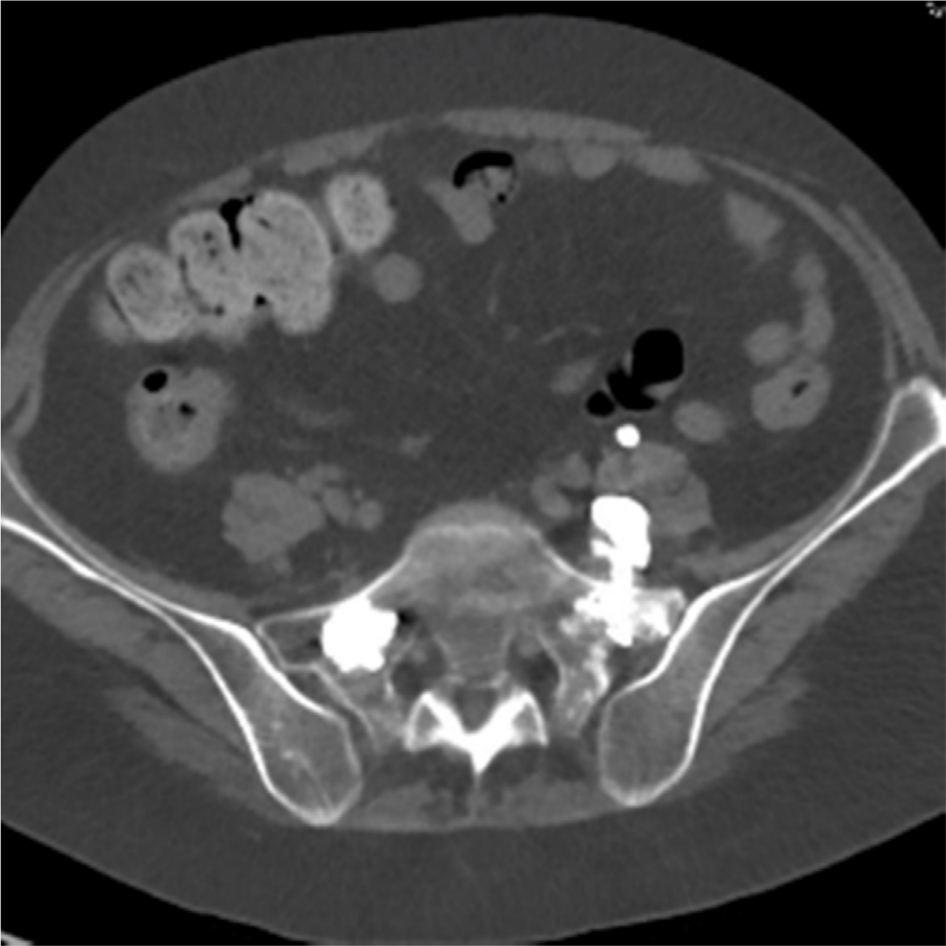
Axial view CT without contrast of lumbosacral spine demonstrating cement extravasation anterior to left sacral ala along the course of left L5 nerve root.
